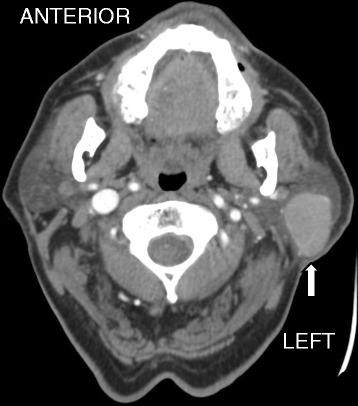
Computed tomography of neck soft tissue demonstrates an enhancing mass (white arrow) within the left parotid gland measuring 3.3 cm (anterior-posterior) × 2.2 cm (transverse) × 3.4 cm (craniocaudal). No enlarged lymph nodes or abnormal fluid collections are seen
